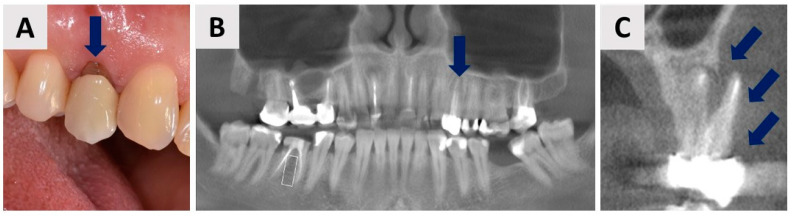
Initial clinical and radiological examinations. (A) Labial view of tooth 24 (FDI notation). (B) Panoramic CBCT section showing radiolucency at the apex of tooth 24 (blue arrow). (C) Coronal CBCT section revealing complete destruction of the buccal bone wall in the region of tooth 24. Blue arrows mark the area where the buccal bone wall previously existed.
